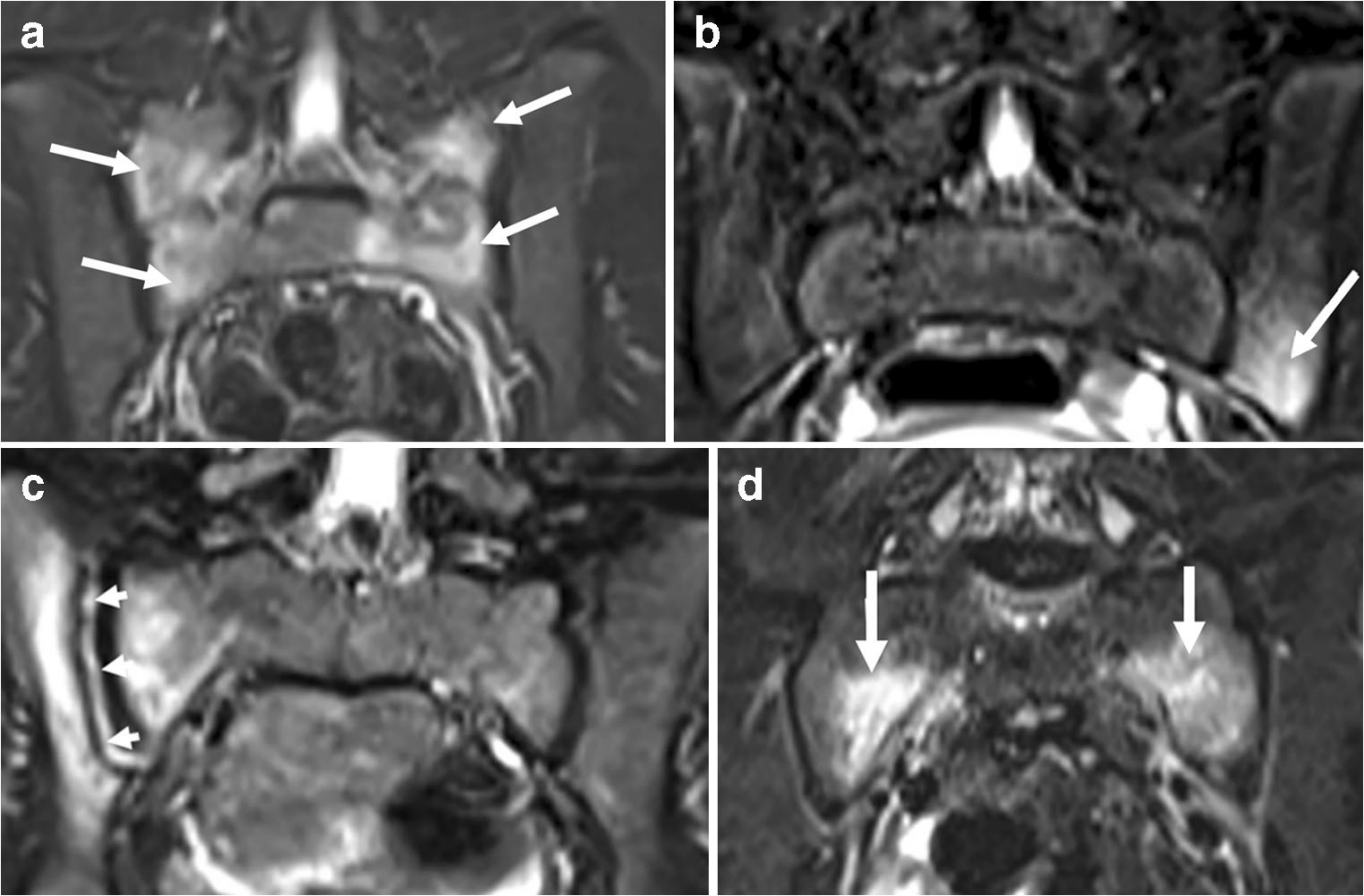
Chronic nonbacterial osteomyelitis with sacroiliac joint involvement in four children aged 10–15 years. Coronal T2-W short tau inversion recovery MR images (repetition time/echo time = 5,000/58 ms). a Bone marrow edema at the sacral side, bilaterally (*arrows*) in an 11-year-old girl. b Bone marrow edema at the left iliac side (*arrow*) in a 9-year-old girl. c High signal in the right joint space *arrows*, with surrounding bone marrow edema at the iliac and sacral sides in an 8-year-old girl. d Bone marrow edema at the sacral sides, bilaterally in a 15-year-old girl (*arrows*)
